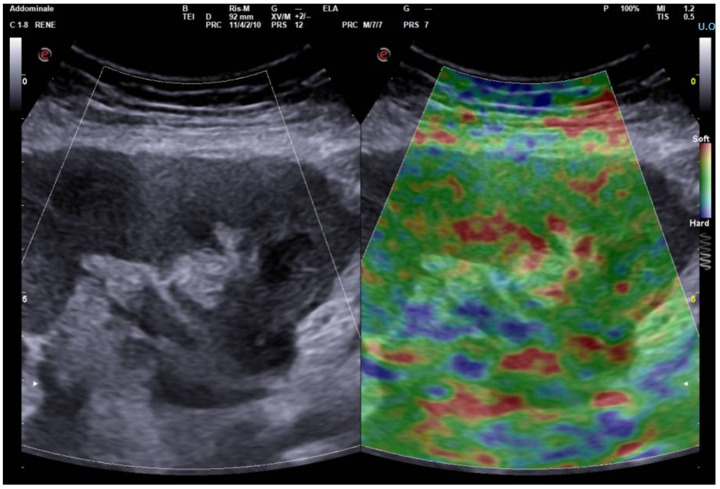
This figure presents a comparative visualization of a colorimetric elastographic map (right) and a traditional B-mode grayscale ultrasound image (left) of the kidney. The colorimetric map represents the spatial distribution of tissue stiffness, with different colors corresponding to varying degrees of elasticity. The reference scale provides a quantitative interpretation of the color-coded stiffness values. While this dual-modality approach offers a visual representation of tissue heterogeneity, it should be noted that the colorimetric map does not provide specific multiparametric information and therefore this visualization technique, when applied to deep tissue studies, may not yield additional data, thus limiting its diagnostic impact.
